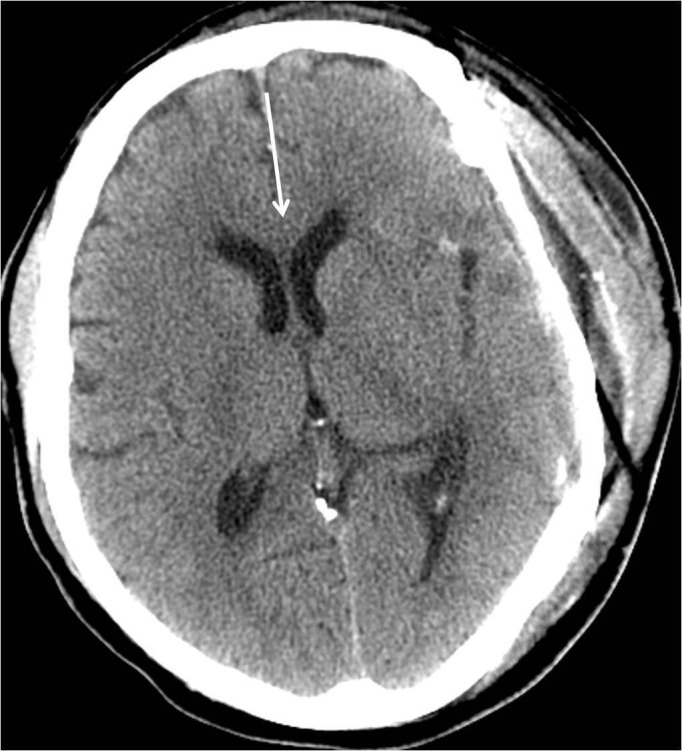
Postoperative head computed tomography (CT) prior to discharge reveals a left sided craniectomy and cranioplasty procedure with resection of the tumor components both intracranial and extracranial. There has been resolution of mass effect and midline shift (arrow).
